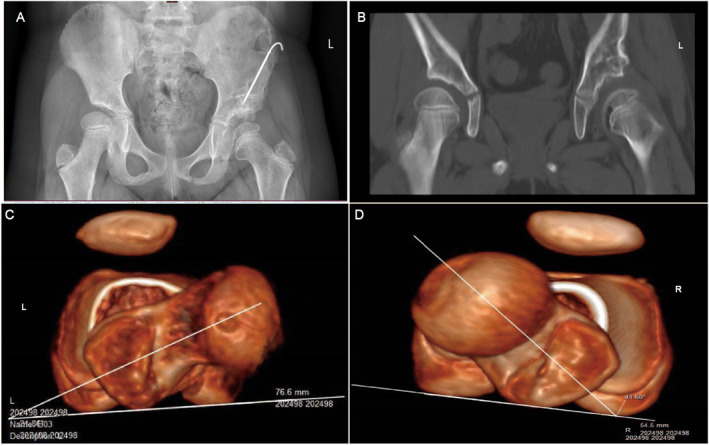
Follow‐up images, 1 year after surgery: anteroposterior radiographs of the pelvis (A) and computed tomography scan of the hip joint showed no lesions (B); 3D reconstruction of the left/right acetabular angle (C, D).
